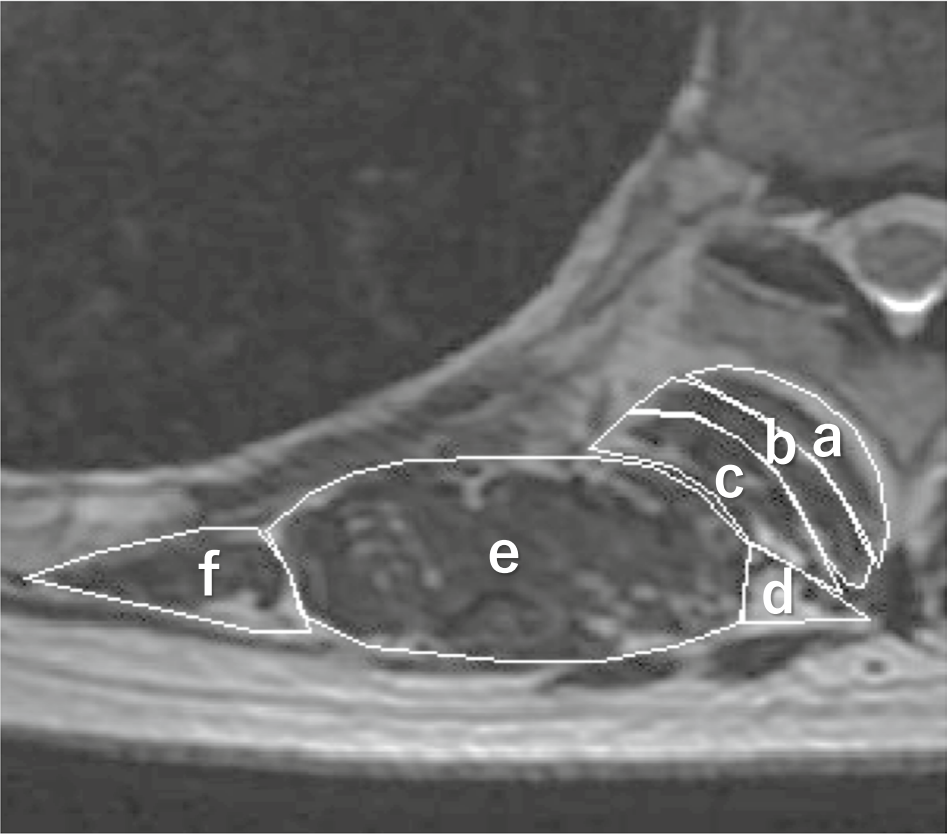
Posterior extensor muscles visualized on an axial T2-weighted image. The cross-sectional area of the transversospinalis muscles (a, rotatores; b, multifidus; c, semispinalis), erector spinae muscles (d, spinalis; e, longissimus; f, iliocostalis) were measured by using the calibrated scale on the MR images.
